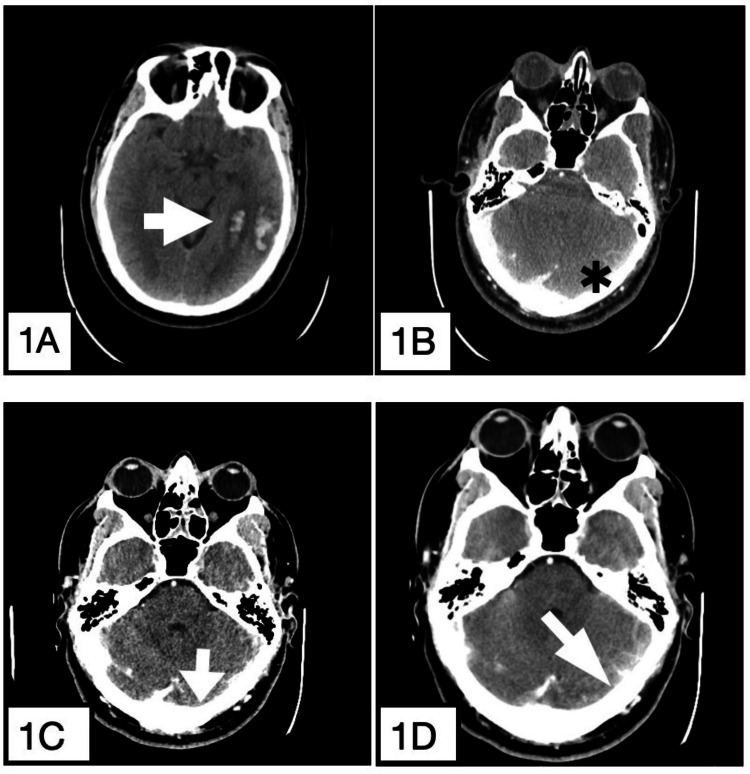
(A) A cranial computed tomography (CT) scan showing two hemorrhagic lesions in the left temporal-occipital territory without underlying vascular lesions (white arrow). (B) Transverse and sigmoid venous sinuses and the left Labbé vein asymmetry (black asterisk). (C) A venous phase extension confirmed that parenchymal hemorrhages were caused by venous infarction after thrombosis of the transverse and sigmoid venous sinuses and the left Labbé vein (white arrow) with (D) the absence of enhancement of those sinuses previously mentioned on the left side. The vein of Labbé is also not identified on the left side.
